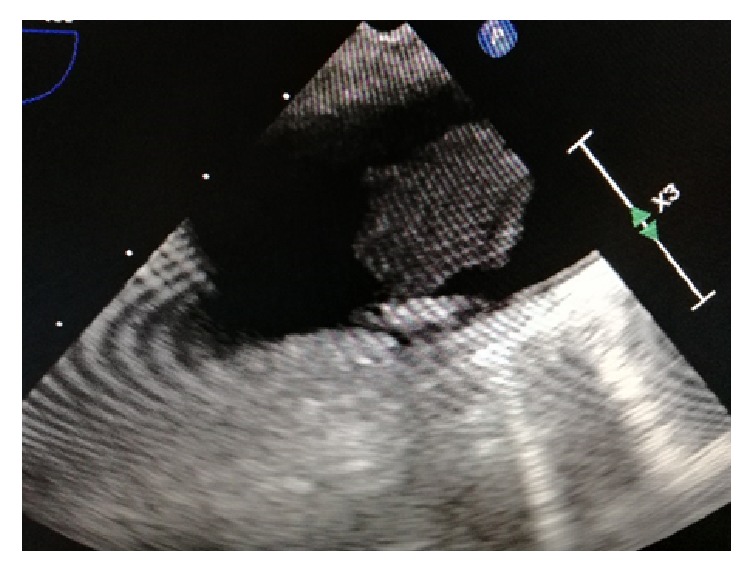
Transesophageal echocardiography showed a 23 mm mobile thrombus in the lesser curvature of aortic arch.
